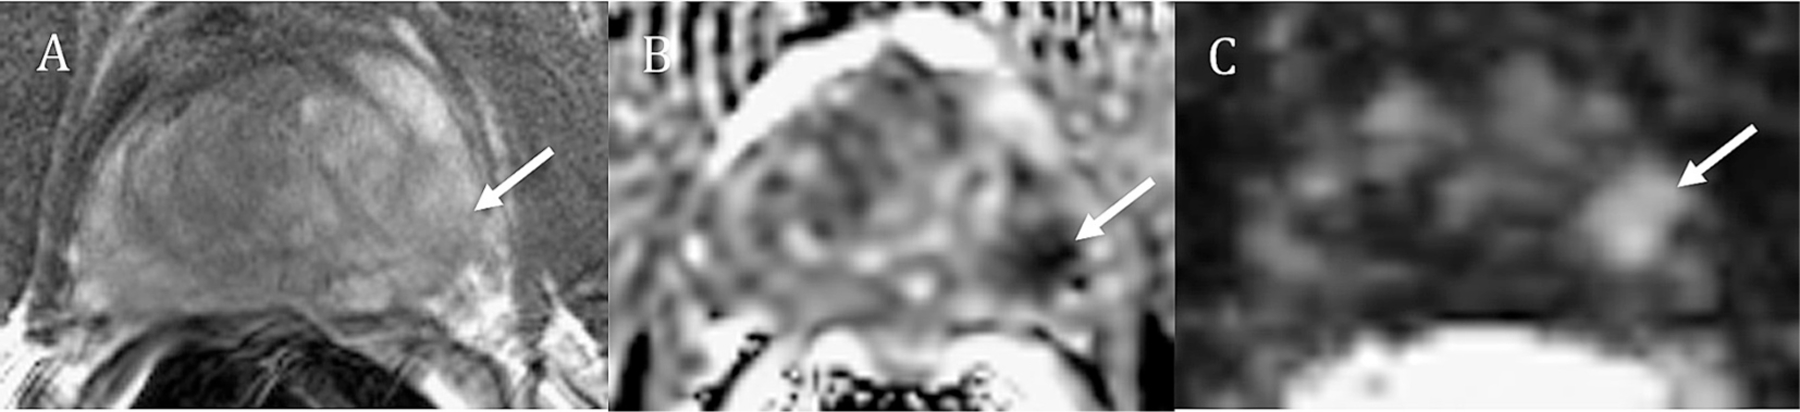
MRI of a 60 year-old male with serum PSA of 9.3 ng/ml who underwent radical prostatectomy. Sparse GS4+5 /GG 5 cancer (20% cancer) as seen on: A) coil-corrected T2-weighted image (normalized T2=1.45), B) ADC map (1114×10–3mm2/s), and C) maximal enhancement slope (149 %baseline/min). The arrows designate the cancerous region.
